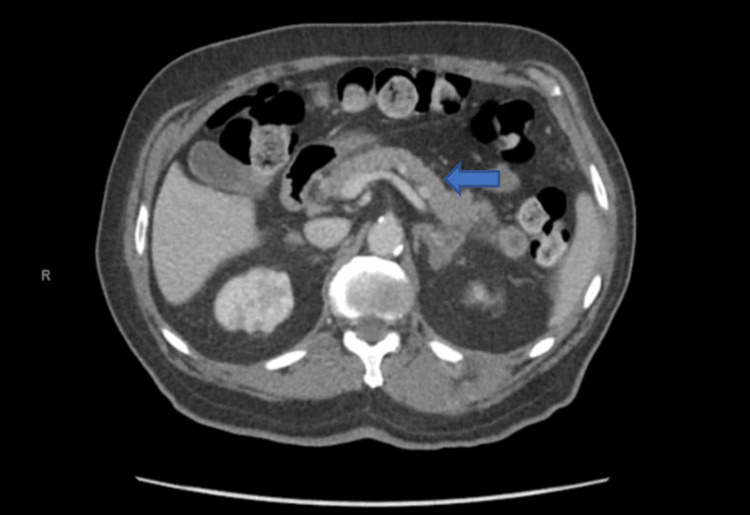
CT scan of the abdomen showing multiple new pancreatic metastases (arrow)
